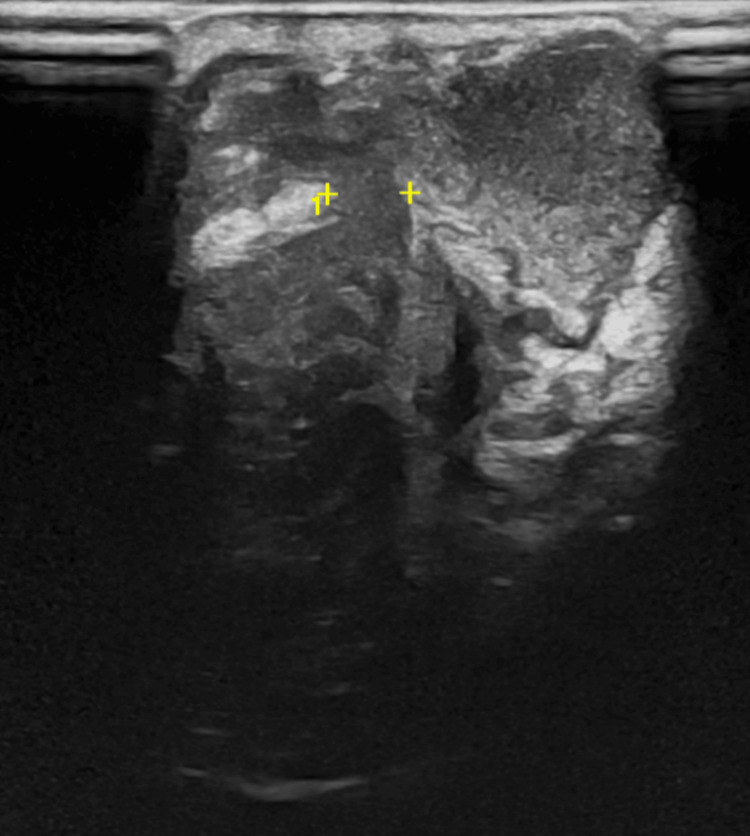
Right wrist ultrasound showing intra-articular extension. Ultrasound view highlighting the tract of the abscess collection extending through the deep planes into the joint and echogenic material filling the joint space.
